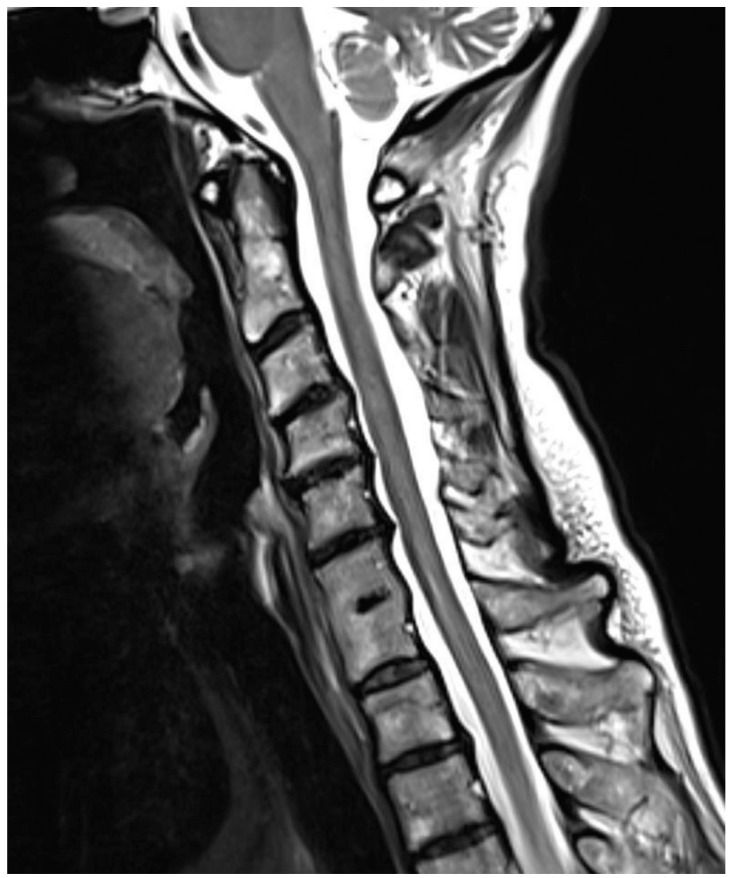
Sagittal T2-weighted fast spin-echo sequence; 59 y/o female; congenital block vertebra C6/7 with degenerative changes in segments C3/4, C4/5, and C5/6 (disk prolapse); however, the segment C7/Th1 is not affected by degeneration, representing a combination of ASDI in one segment and natural degenerative changes. The degeneration score in this patient, who has degeneration in segments C3/4 and C4/5 but not in segments C1/2 and C2/3, is 2 (1 point per segment for loss of height of the intervertebral disk, disk bulging over the dorsal level, and evidence of retrospondylophytes). The respective degeneration ratio is 0.167 (2/12). The degeneration score for the adjacent segments in this patient, who has degeneration in segment C5/6 but not in segment C7/Th1, 1 (loss of height of the intervertebral disk, disk bulging over the dorsal level, and evidence of retrospondylophytes). The adjacent segment ratio is 0.167 (1/6). Consecutively, the instability ratio is 0 (0.167–0.167), which means that the natural degeneration and the degeneration caused by the block vertebra are equal.
